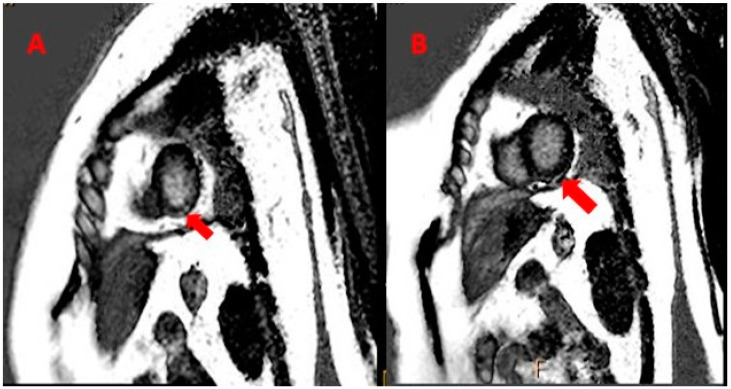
MRI of the heart, showing LGE in inferior lateral left ventricular (red arrows) (A). In addition, minimal pericardial effusion was noted (red arrows) (B).
